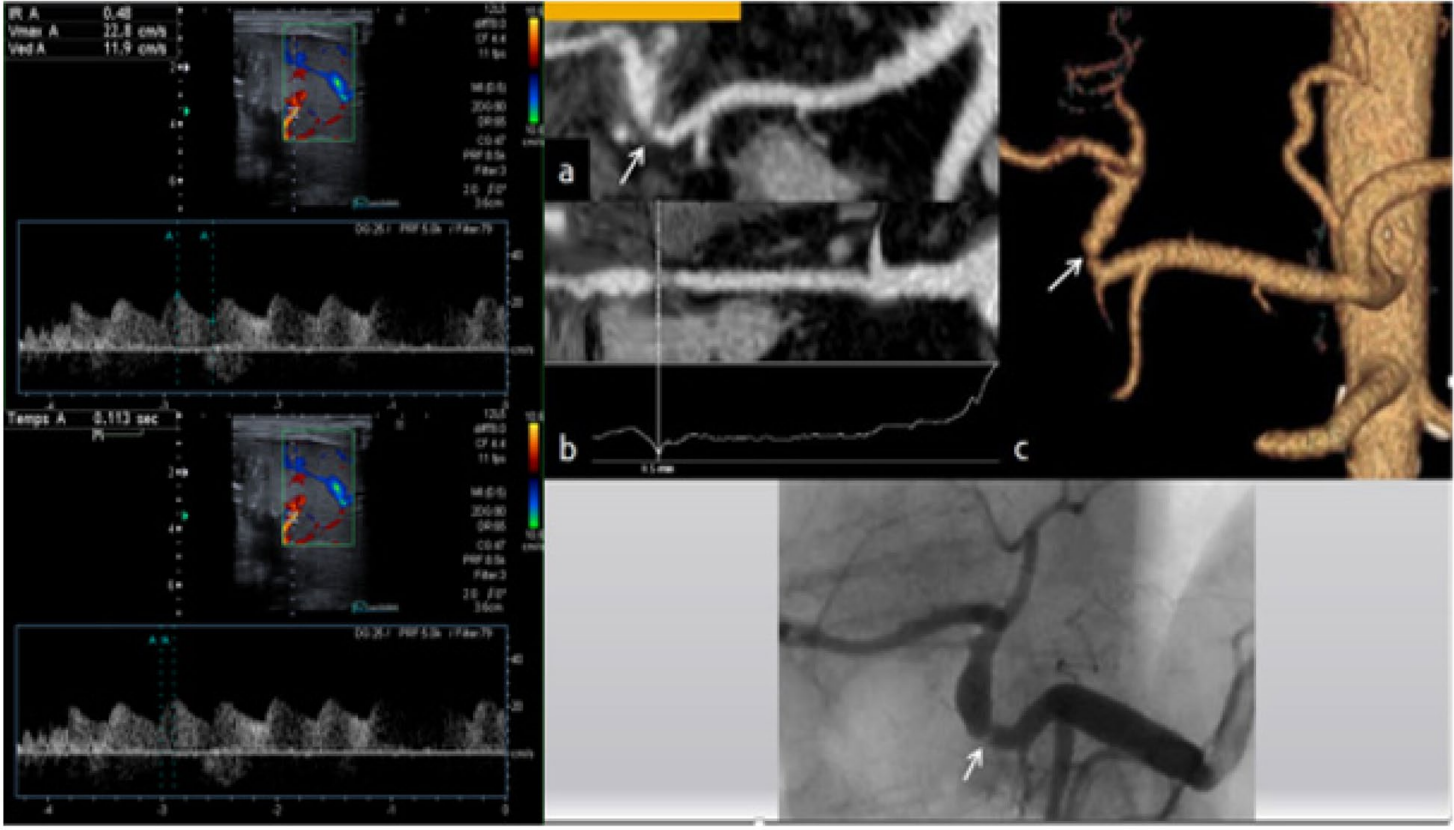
Typical tardus parvus (IR = 0,48, TAS =0,11 s) and confirmation of a significant arterial stenosis with angio CT and angiography
